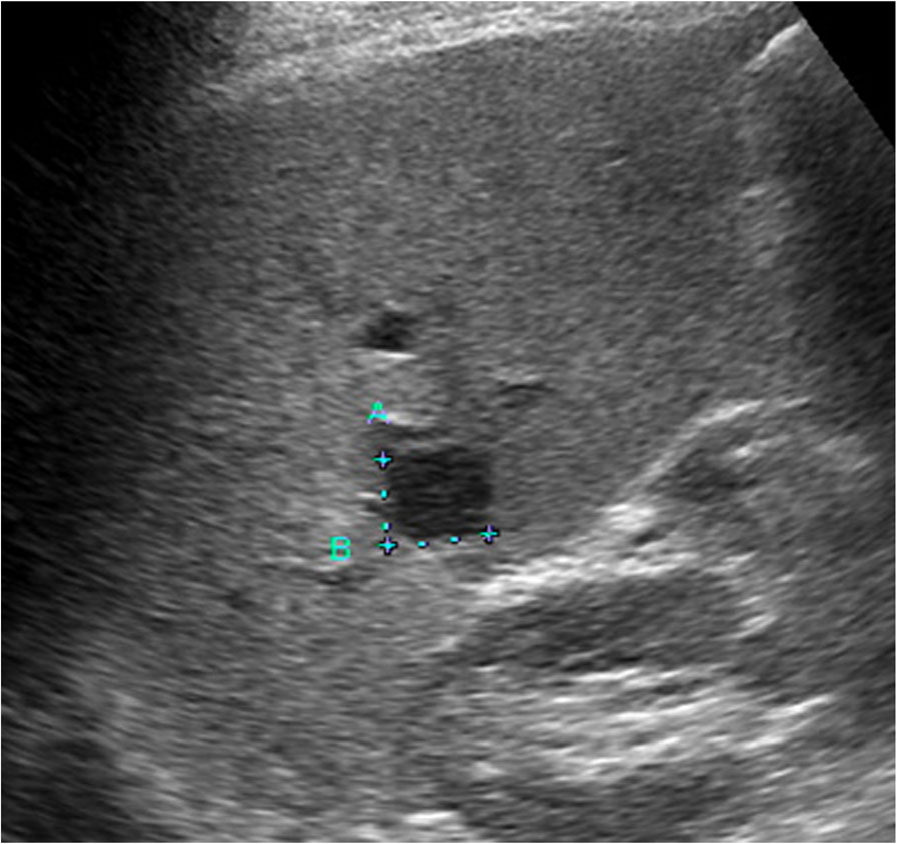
Abdominal ultrasound reveals a well-demarcated, homogenous, hypoechoic round nodule in segment 6 of the liver
